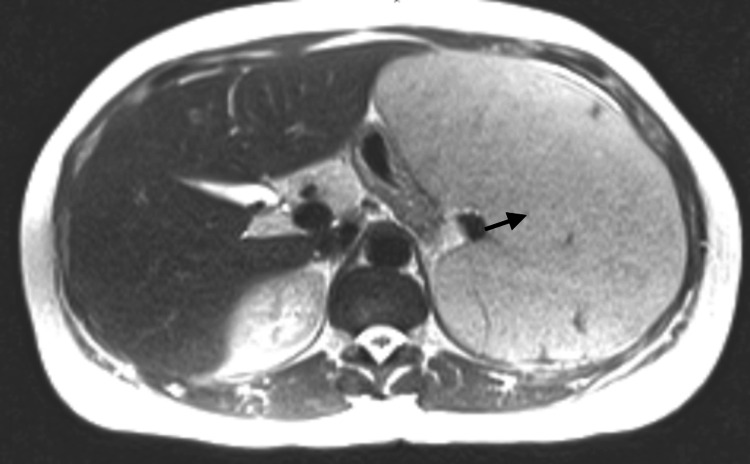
MRI abdomen with contrast showing massive splenomegaly (marked by a black arrow)
